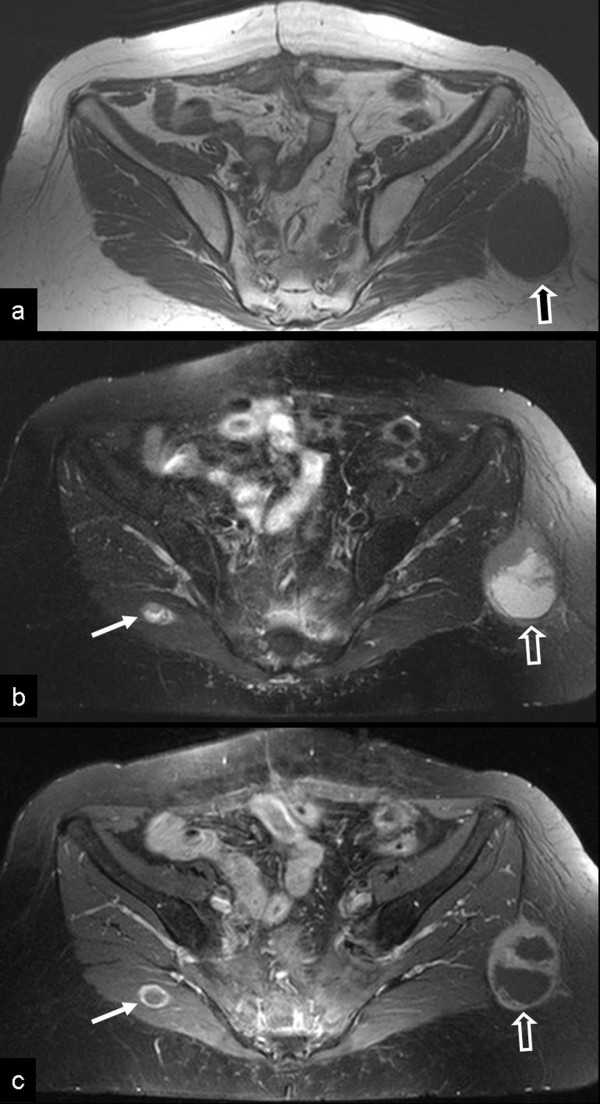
Metastatic disease from extragastrointestinal GIST. a) The axial T1-w MR image shows an intermediate signal intensity ovoid-shaped mass, lateral to the gluteus maximus muscle, within the subcutaneous fat (arrow). An intact fat plane separated the mass from the muscle. b) The fat suppressed T2-w TSE MR image, shows the high signal intensity of the central mass and the intermediate signal intensity of the anterior wall (open arrow). A second smaller lesion with similar imaging characteristics is shown in the right gluteus maximus muscle (thin arrow). c) The contrast enhanced fat suppressed T1-w SE MR image shows the peripheral enhancement of the wall (open arrow). The central non enhancing component presumably corresponds to necrosis. Ring-like enhancement is also shown in the smaller lesion (thin arrow).
